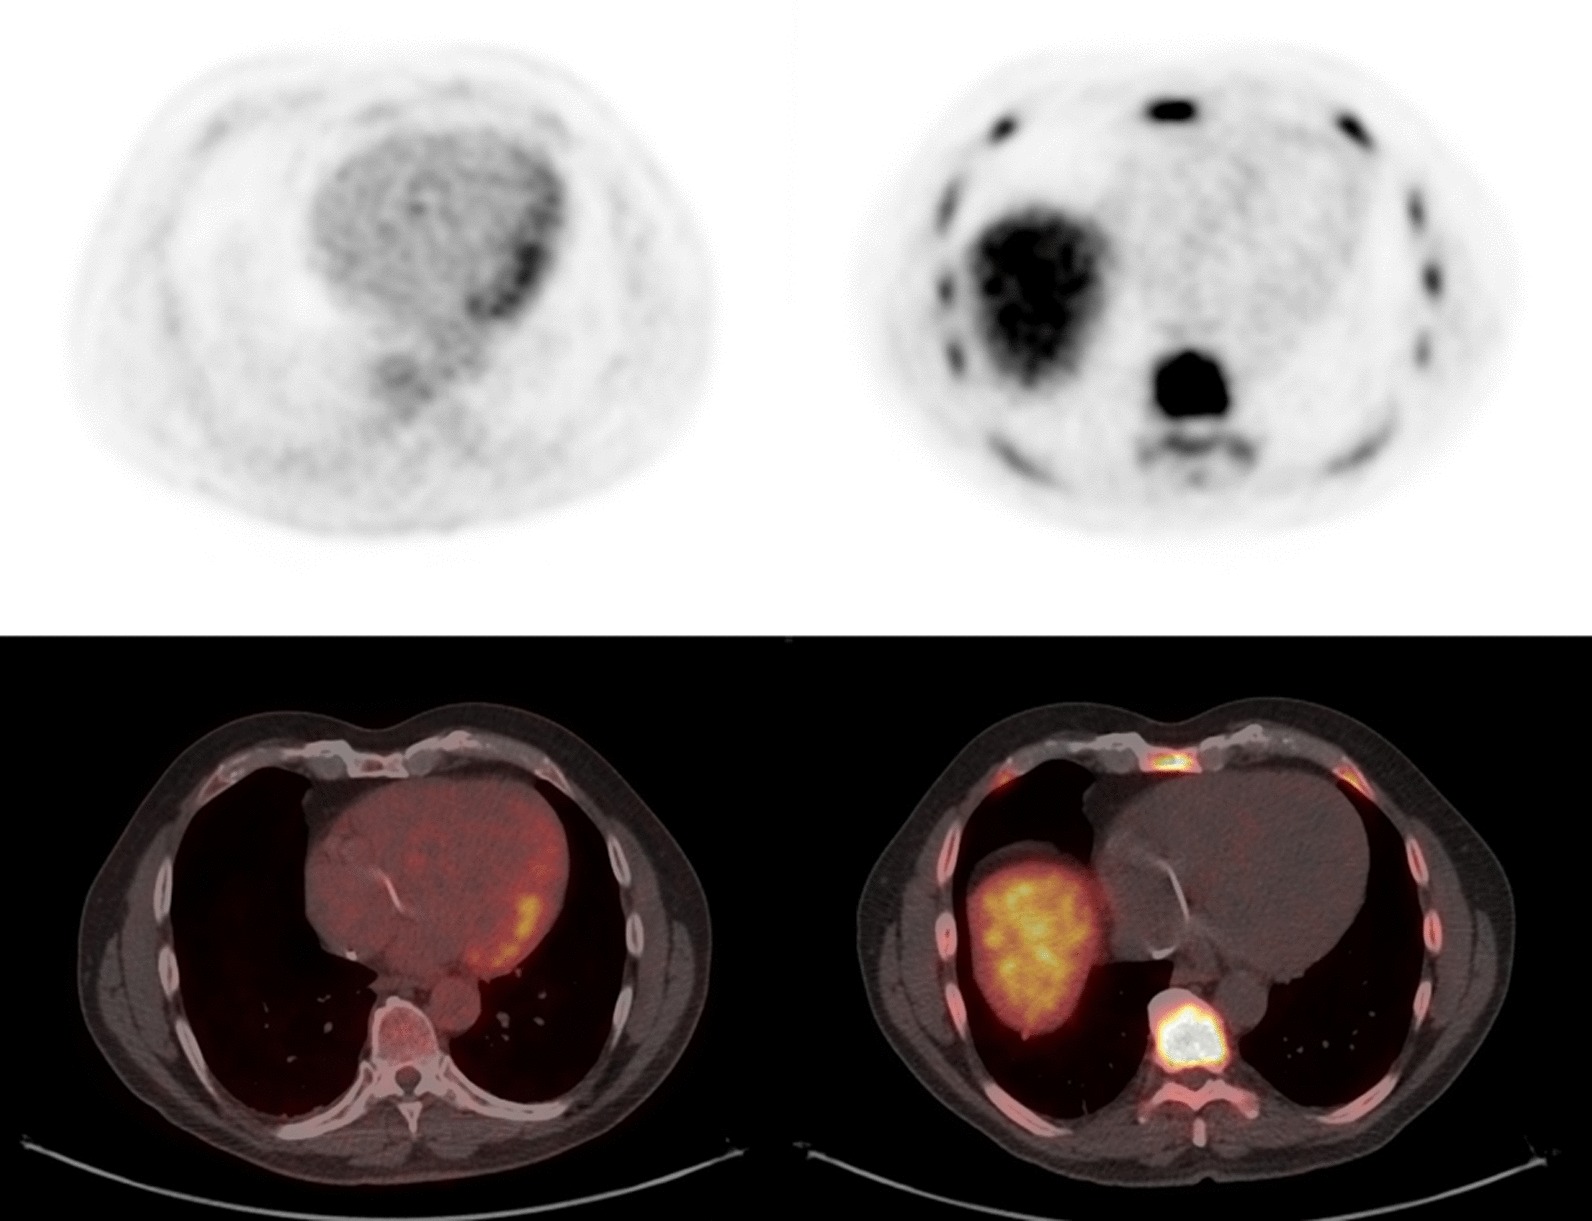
Subject demonstrating isolated diffuse lateral ventricular wall FDG uptake (left), a finding usually interpreted as a normal variant. No corresponding uptake was present on the FLT study (right)
